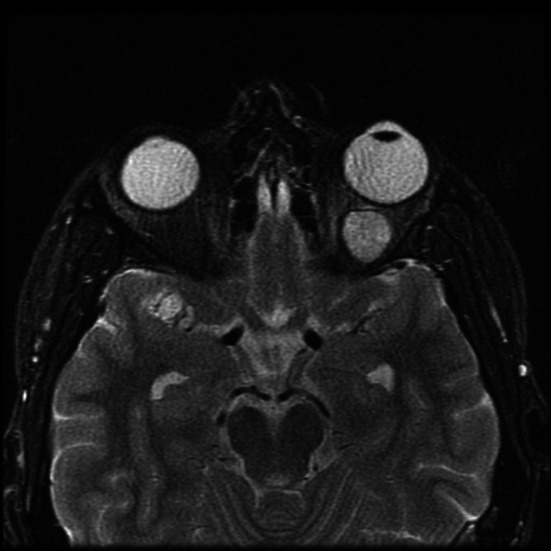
MRI from 2008, dimensions 18 × 14 mm
